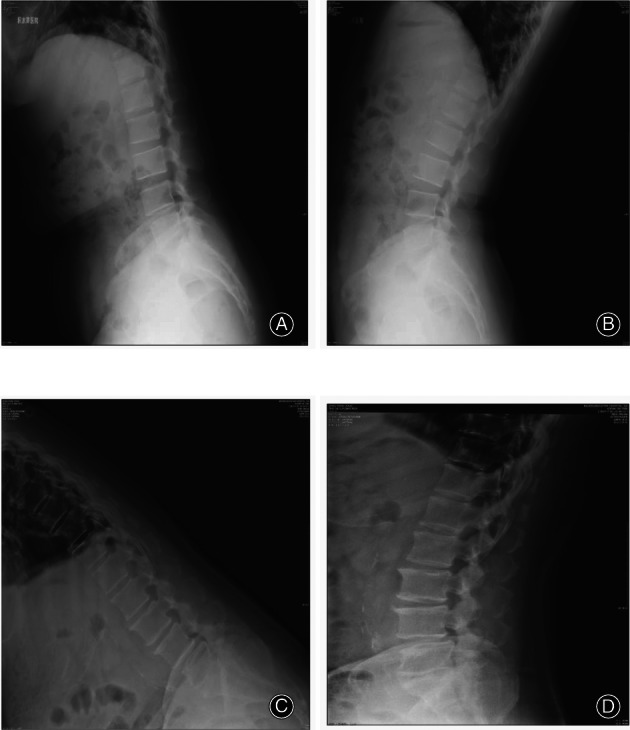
(A) Flexion radiograph of case 1 (MI‐TLIF). (B) Extension radiograph of case 1 (MI‐TLIF). It can be seen that the L4/5 joint gap becomes larger. (C) Flexion radiograph of case two (OLIF). (D) Extension radiograph of case 2 (OLIF). Spondylolisthesis of Meyerding grade 1 (slippage ≤25%) at the L4–L5 level was confirmed by radiography
